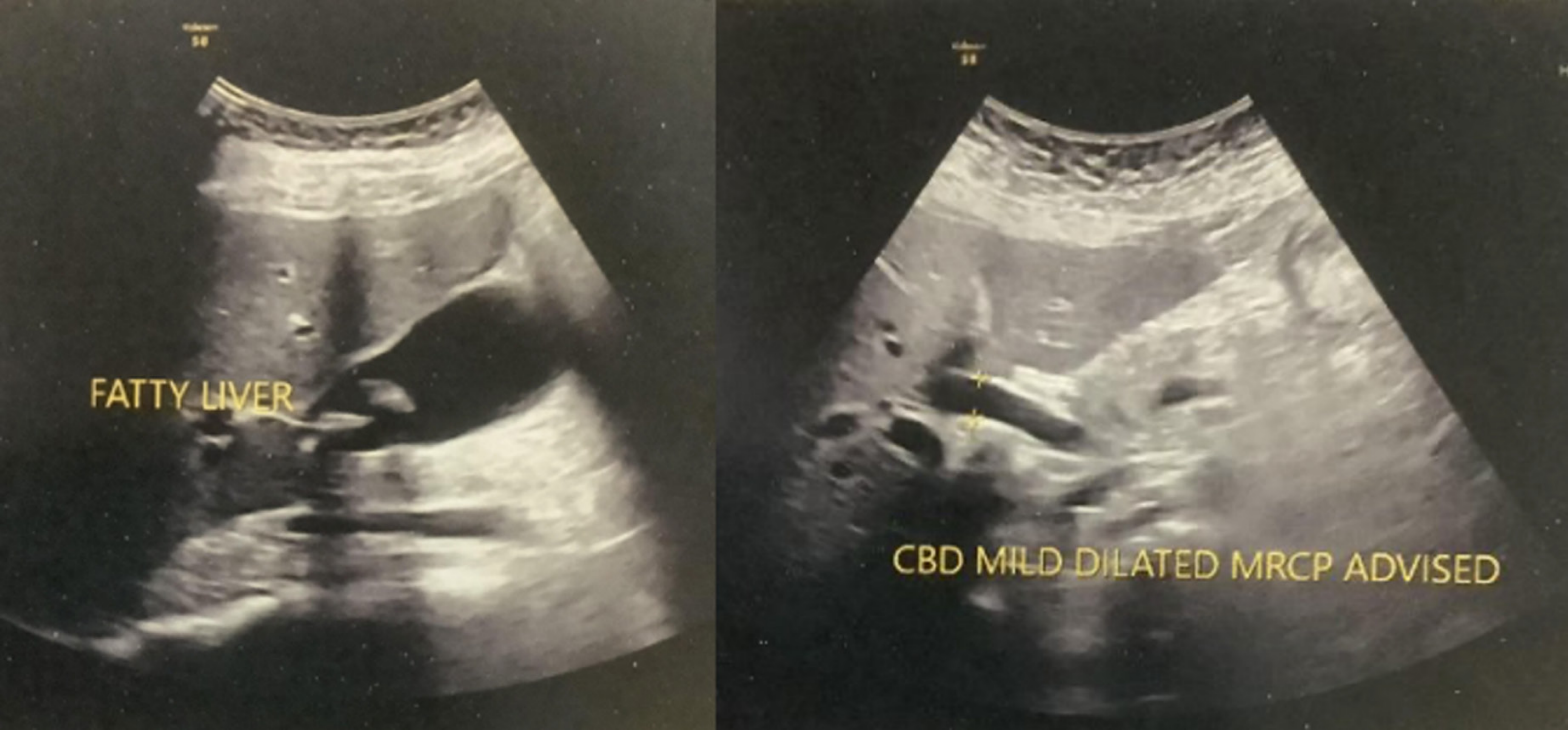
Ultrasonography scans of the patient.
